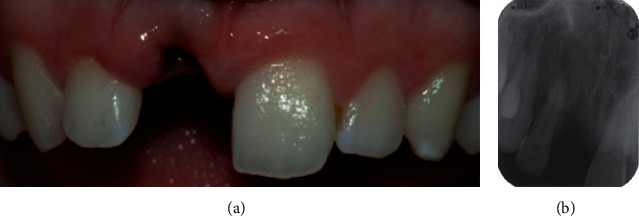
Posttraumatic (a) clinical and (b) radiographic view showing the absence of the upper right central incisor.
